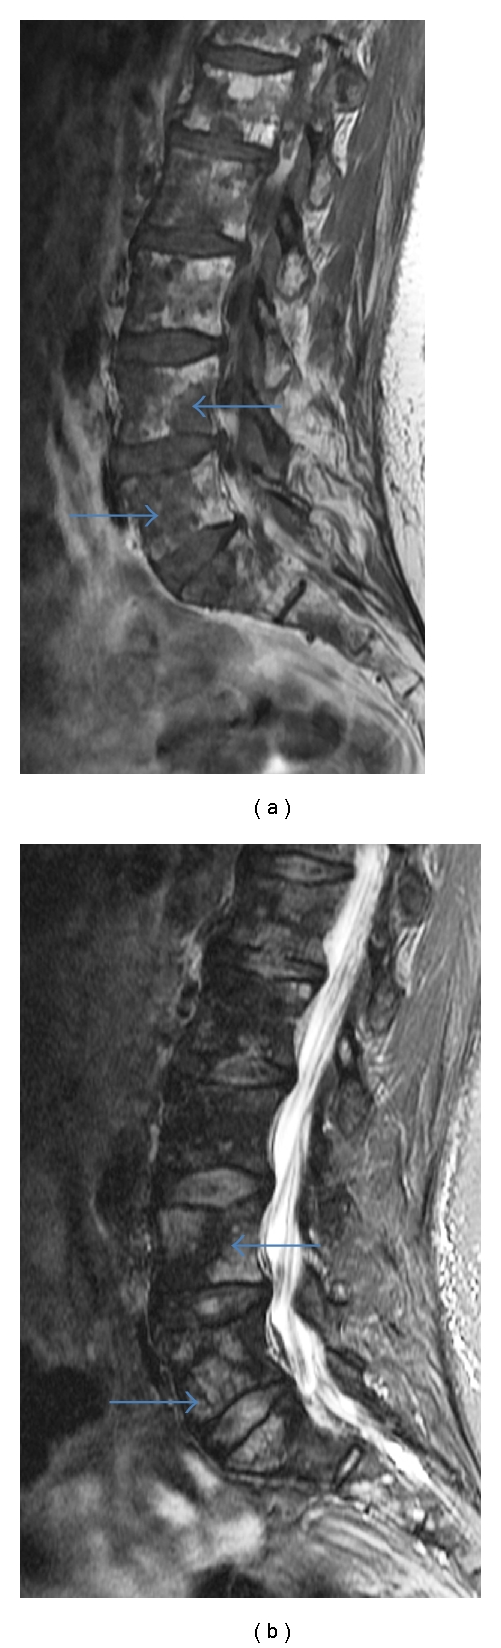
(a) MRI sagittal T1-weighted sequence lumbar spine: diffuse permeative low signal myelomatous marrow lesions throughout the lumbar spine (arrow). (b) MRI sagittal T2-weighted STIR sequence (same patient): diffuse high signal myelomatous marrow lesions throughout the lumbar spine (arrow).
